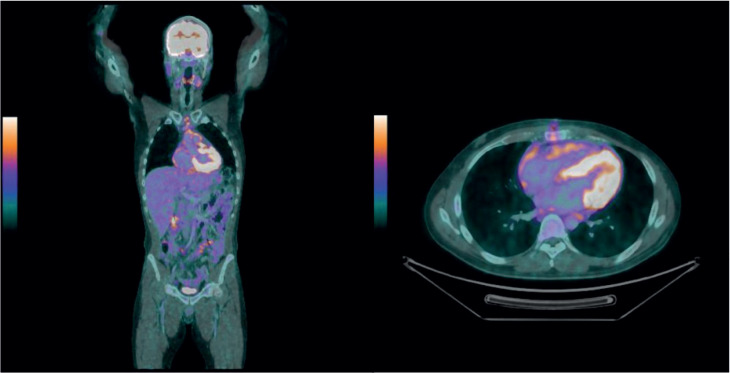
FDG PET positive for a solid lesion in the superior pericardial recess, extending to the myocardium (anterior wall of the left ventricle), as well as multiple solid nodules diffusely distributed in the pericardium and mediastinal lymph nodes with hypermetabolism.
